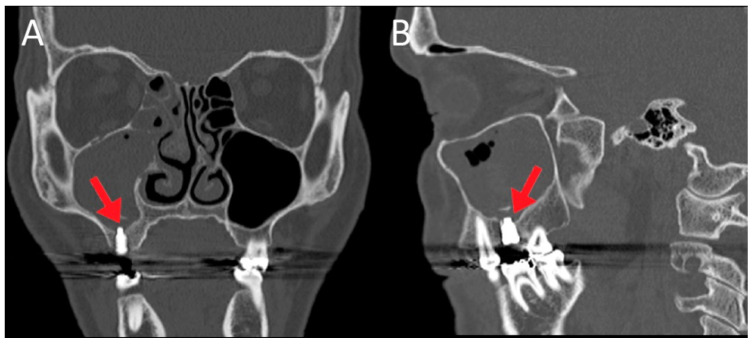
Female patient, 64 years old, who had undergone dental implant surgery 3 months earlier, complaining of left facial pain. CT (A): coronal scan; (B): sagittal scan) revealed odontogenic right maxillary sinusitis (red arrows indicate the dental implant). No migration of the dental implant was documented. An endoscopic primary surgical approach was proposed. The removal of implant removal was not necessary.
